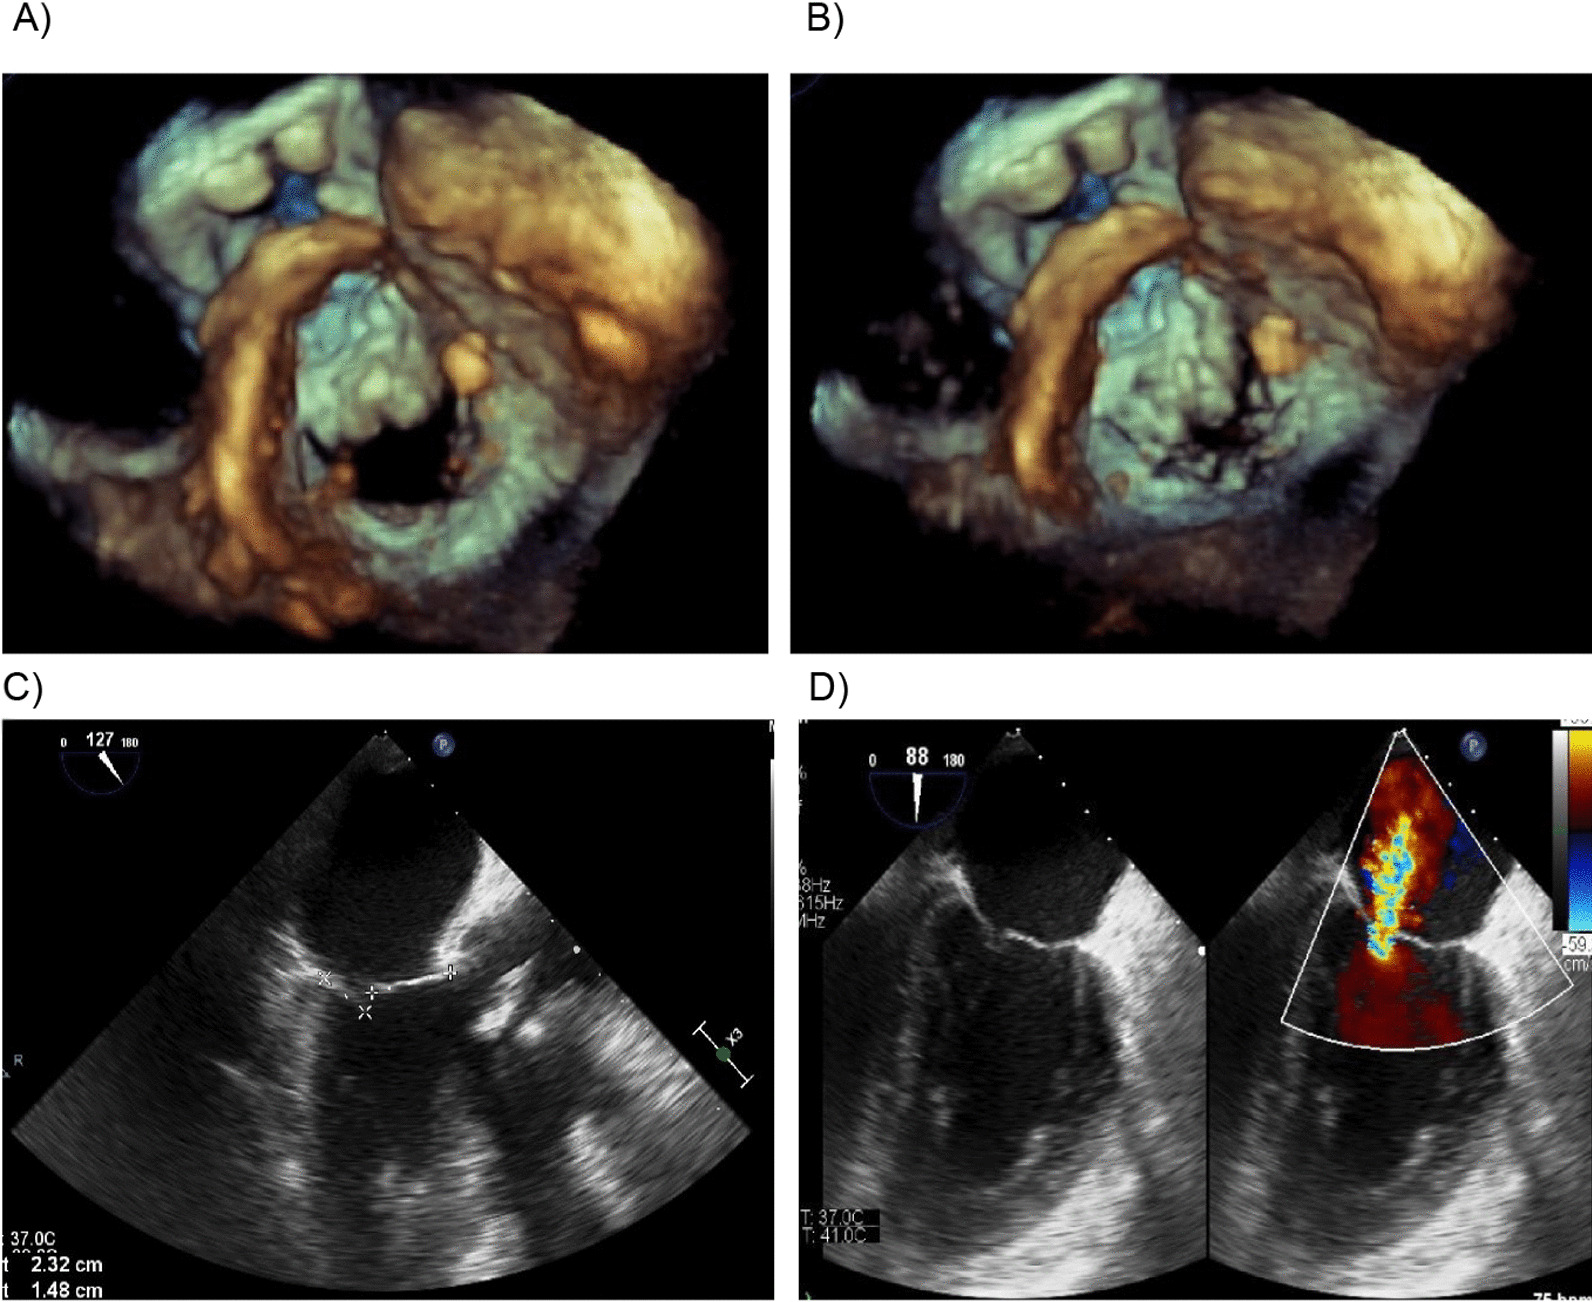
Echocardiogram images after SU AVR using traditional sutured stented aortic prosthesis. A Mitral valve orifice—diastolic opening. B Mitral valve dysfunction during systolic leaflet closure, loss of coaptation. C Slightly restrictive anterior leaflet, impaired apposition and loss of coaptation of the mitral valve. D Severe mitral regurgitation showed by color Doppler echocardiography
